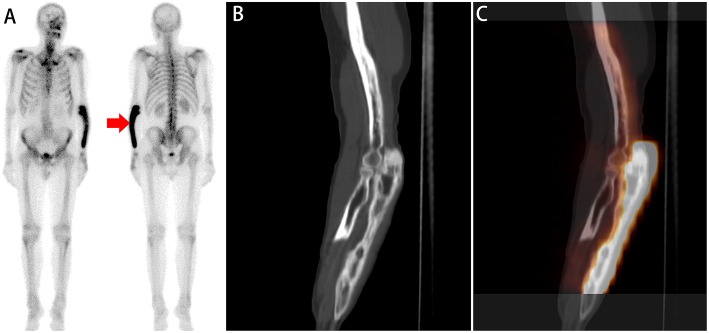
A whole-body scan (A) revealed diffuse high ^99m^Tc-MDP activity in the left forearm (red arrow). No additional areas of abnormal ^99m^Tc-MDP uptake were identified in the remainder of the skeleton. Sagittal (B,C) CT and SPECT/ CT imaging showed diffusely increased ^99m^Tc-MDP uptake corresponding to irregular cortical thickening and porous sclerosis in the ulna. The diagnosis of Paget's disease of bone was established by a combined assessment of clinical and radiologic follow-up.
